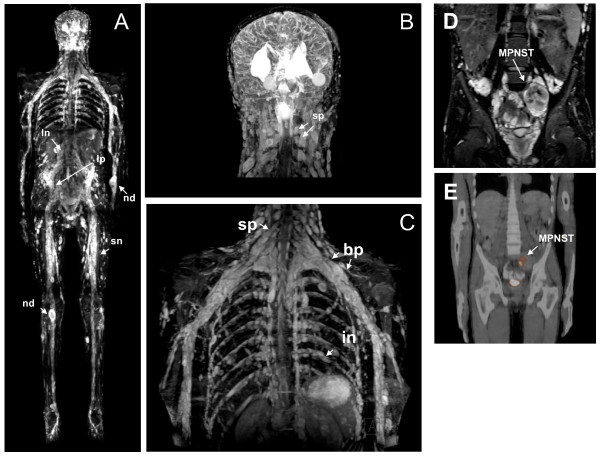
Internal tumor load of our second patient. Whole body MRI (A) indicated multiple tumors along the lumbar nerves (ln), the sciatic nerves (sn) and within the lumbar plexus (lp). Nodular neurofibromas (nd) were also detected. A coronal image of his head (B) indicated multiple spinal neurofibromas (sp), which were also detected on MRI images of his trunk (C) as well as neurofibromas within the brachial plexus (bp) and along the intercostal nerves (in). MRI also showed a high tumor density in the lesser pelvis of our patient (D), including a malignant peripheral nerve sheath tumor (MPNST) as confirmed by positron emission tomography - computed tomography (PET-CT) (E).
